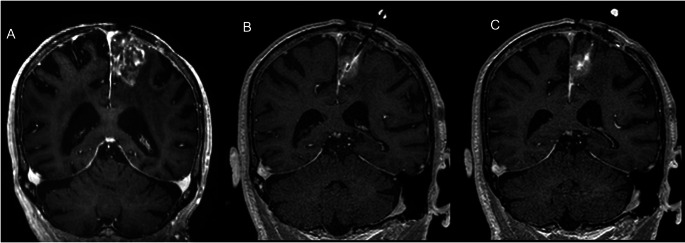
75 year-old man with left superior frontal gyrus GBM recurrence (CED13) demonstrated by enhancing mass on oblique coronal T1-weighted MPRAGE image (A). During CED, the infusate volume grows (B) until backflow is seen along the cannula and its tract (C), with tumor coverage of 12%
